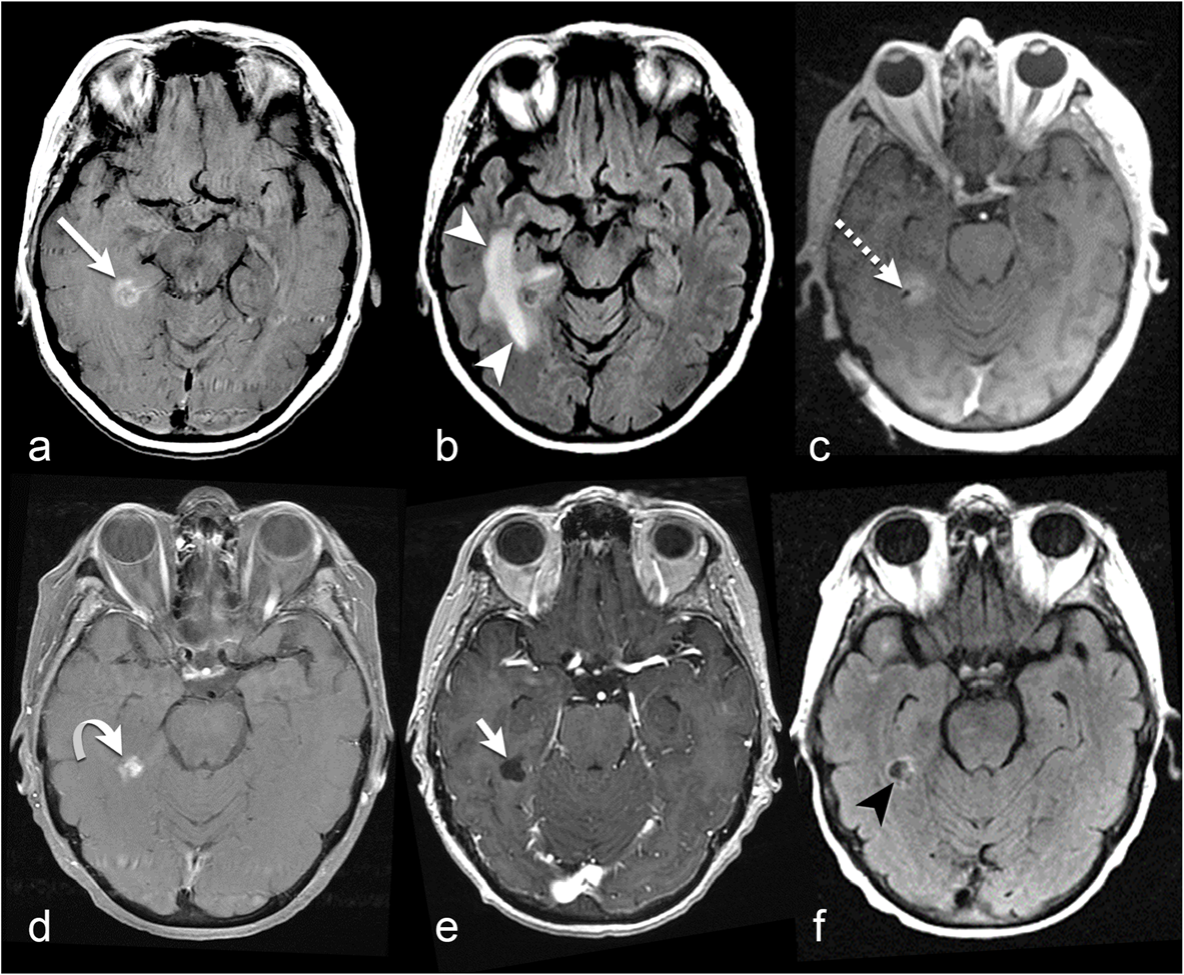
67-year-old with metastatic renal cell carcinoma. a Axial post-contrast T1-weighted imaging before LITT demonstrates a ring-enhancing metastasis in the right medial temporal lobe (long arrow). b FLAIR before LITT demonstrates surrounding vasogenic edema (white arrowheads). c Axial post-contrast T1-weighted imaging shows the ablation probe tip within the metastatic lesion (dashed arrow). d Axial post-contrast T1-weighted imaging 4 months after LITT demonstrates slightly decreased enhancement at the treated lesion (curved arrow). Axial post-contrast T1-weighted imaging (e) and FLAIR (f) 10 months after LITT show complete resolution of enhancement (short arrow) and vasogenic edema (black arrowhead)
